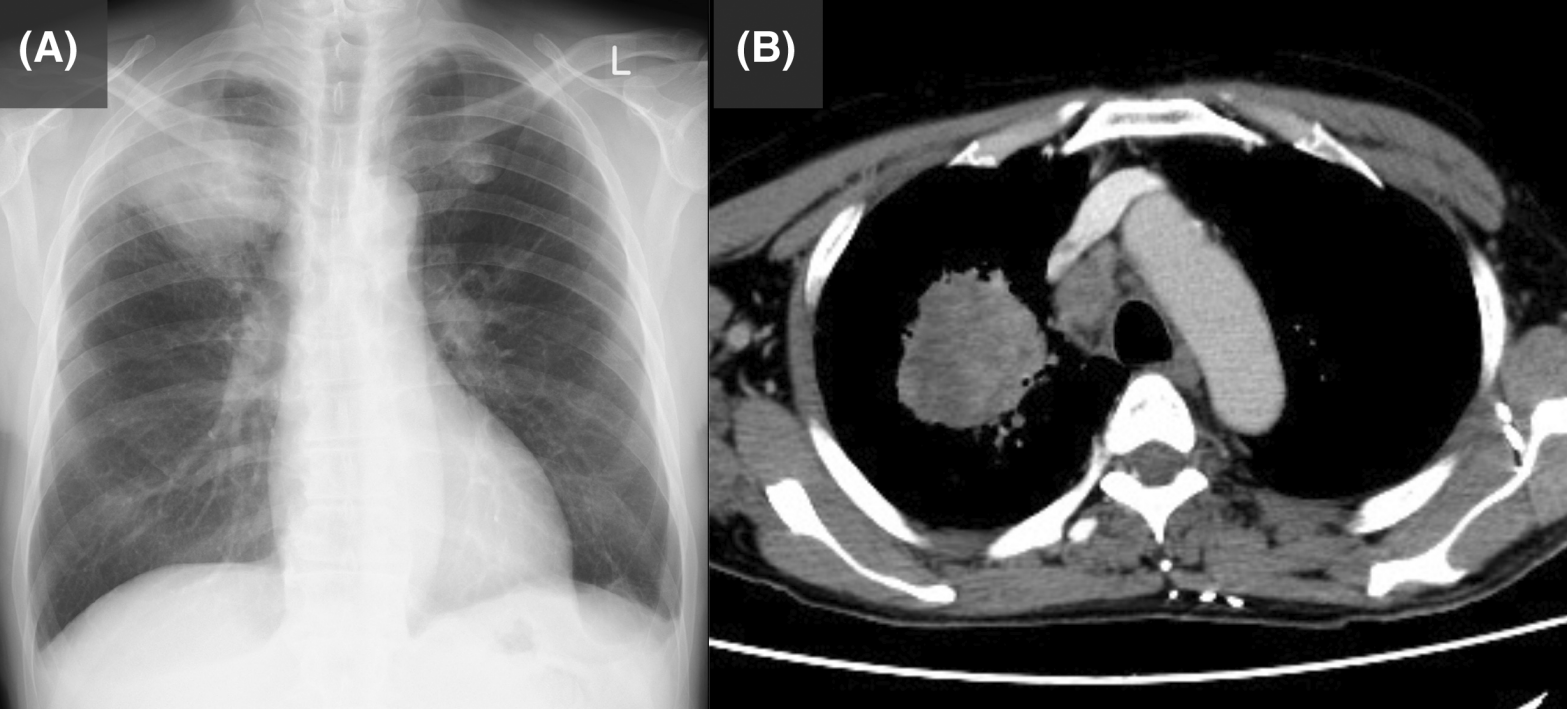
x‐Ray and enhanced computed tomography of the chest. (A) A large tumour is observed in the right upper lung field in the x‐ray. (B) Chest enhanced computed tomography showing a tumour lesion in the right upper lobe and enlargement of the mediastinal lymph node #4R.
